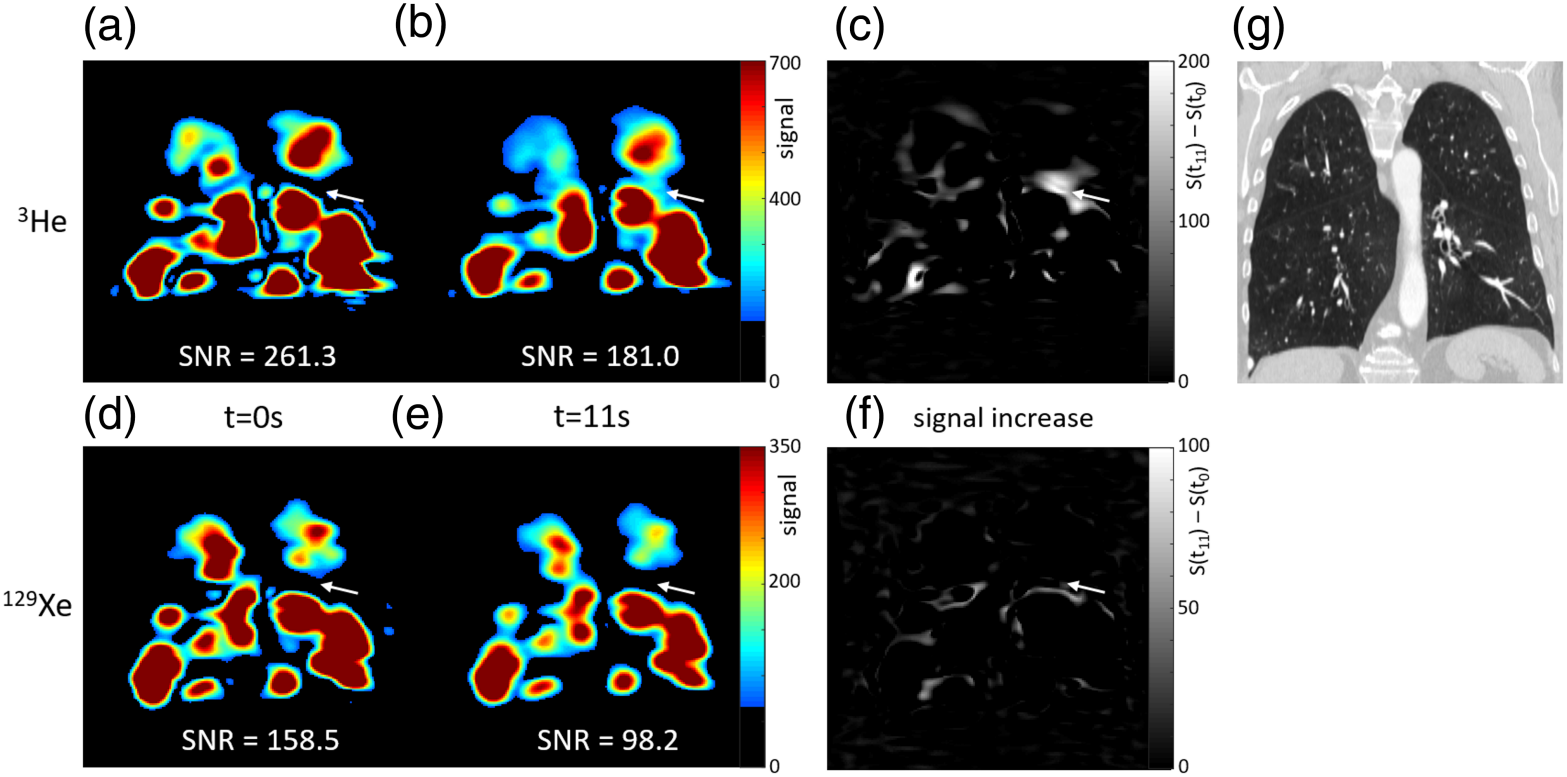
^3^He images (top) and ^129^Xe images (bottom) acquired from patient 1 during breath‐hold; (a,d) at the start of the imaging sequence, t = 0 sec, and (b,e) after 11 seconds, both shown with the same signal range. (c,f) Maps of signal increase from t = 0 sec to t = 11 sec. White arrows highlight a region of lung where ^3^He signal increased over time, but ^129^Xe signal did not. The coronal unenhanced thoracic CT (g) showed moderate centrilobular emphysema and hyperinflation, and an intact left oblique fissure. Mean signal‐to‐noise ratio (SNR) over the whole lung ventilated volume for that timepoint is displayed for a, b, d, and e.
